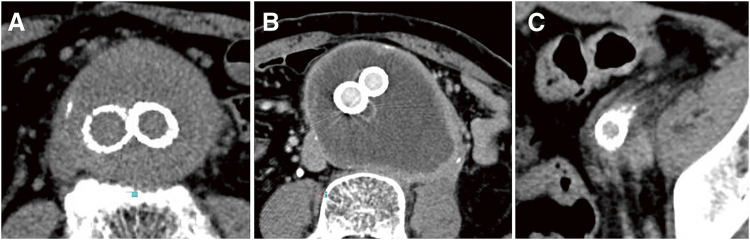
Fig. 1 CT images of the peri-aortitis after EVAR. (A) Soft tissue mass surrounding the aorta. (B) Mild aortic wall thickening with contrast enhancement. (C) Feathery appearance around the aorta. EVAR: endovascular aneurysm repair
